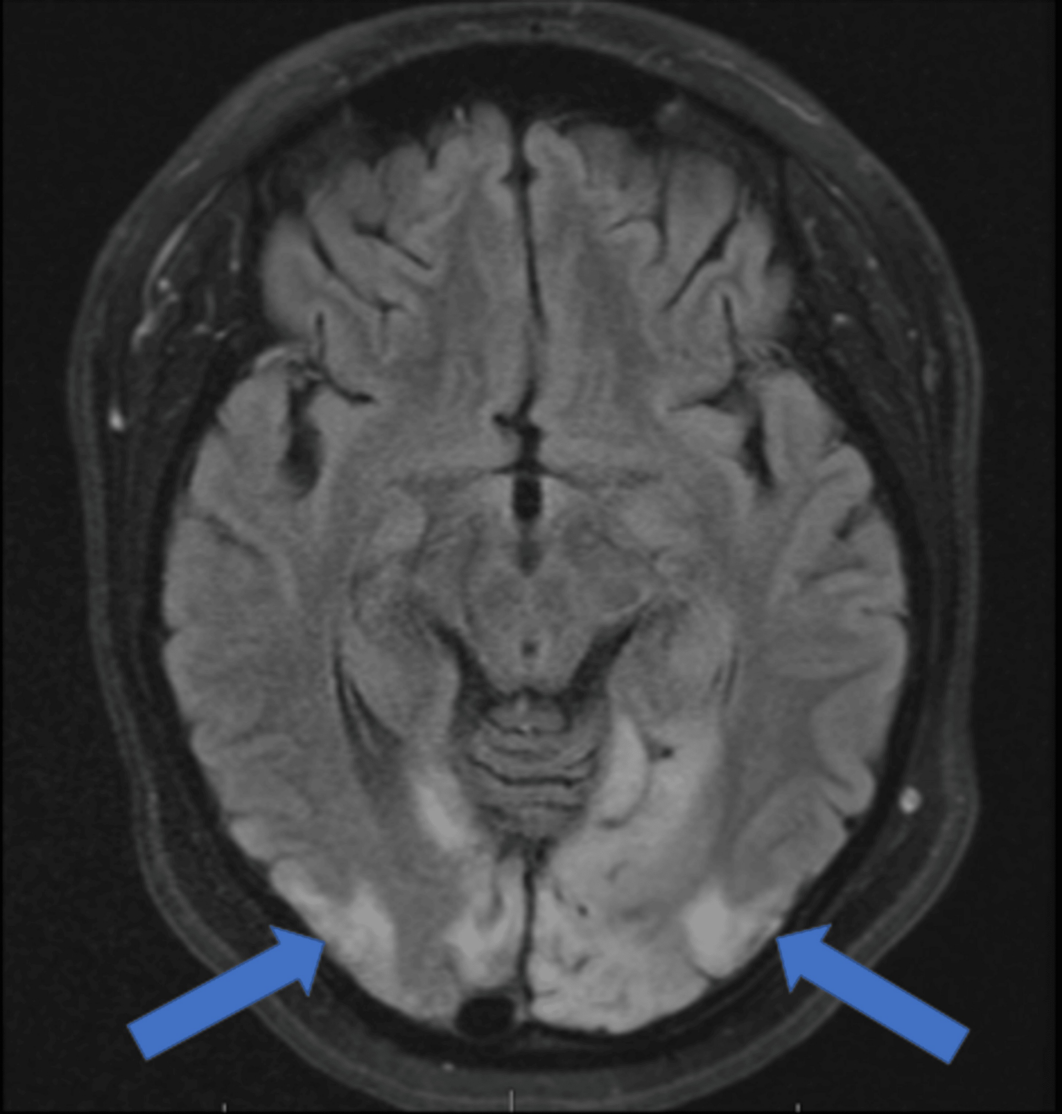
Axial fluid-attenuated inversion recovery (FLAIR) brain MRI. The image demonstrates cortical and subcortical hyperintensities (indicated by arrows) predominantly in the bilateral occipital lobes, more marked on the left, consistent with a stroke-like episode. These findings are characteristics of mitochondrial encephalopathy and do not conform to vascular territory.
